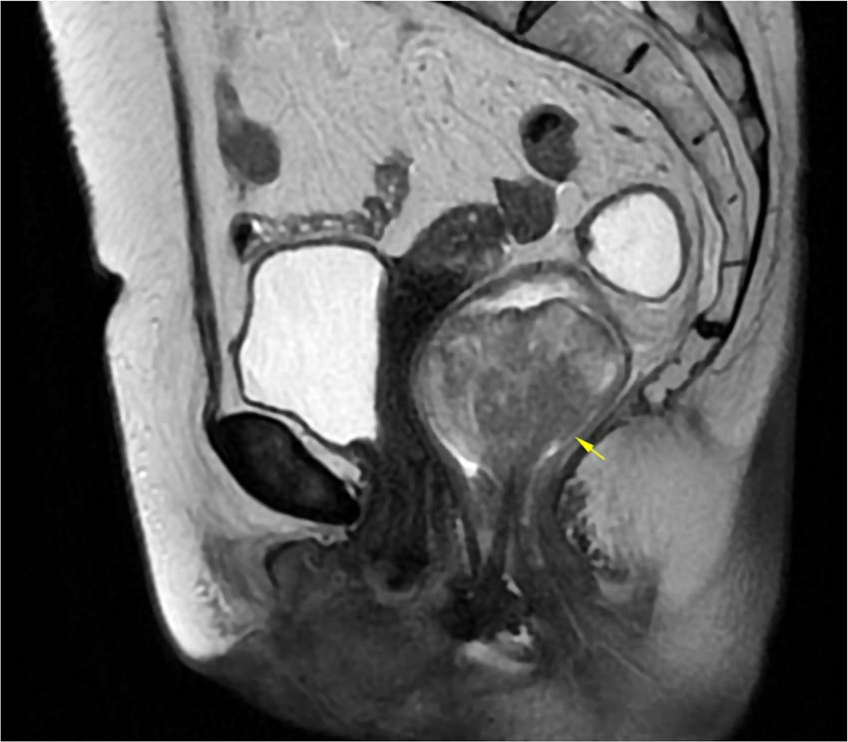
MRI of Case 1 shows a well-defined rectal tumor without regional lymph node involvement. The lesion demonstrates high signal intensity on sagittal T2-weighted imaging (indicated by the arrow).
